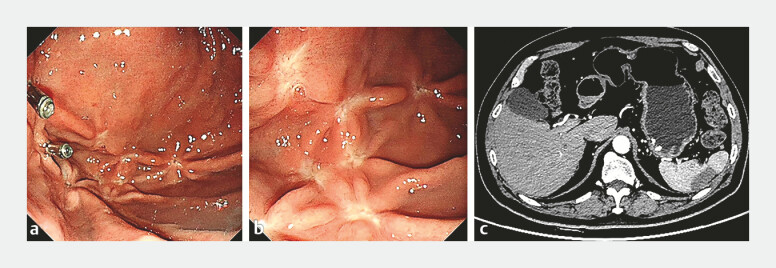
Gastroscopy and computed tomography (CT) scan a month after endoscopic treatment.
